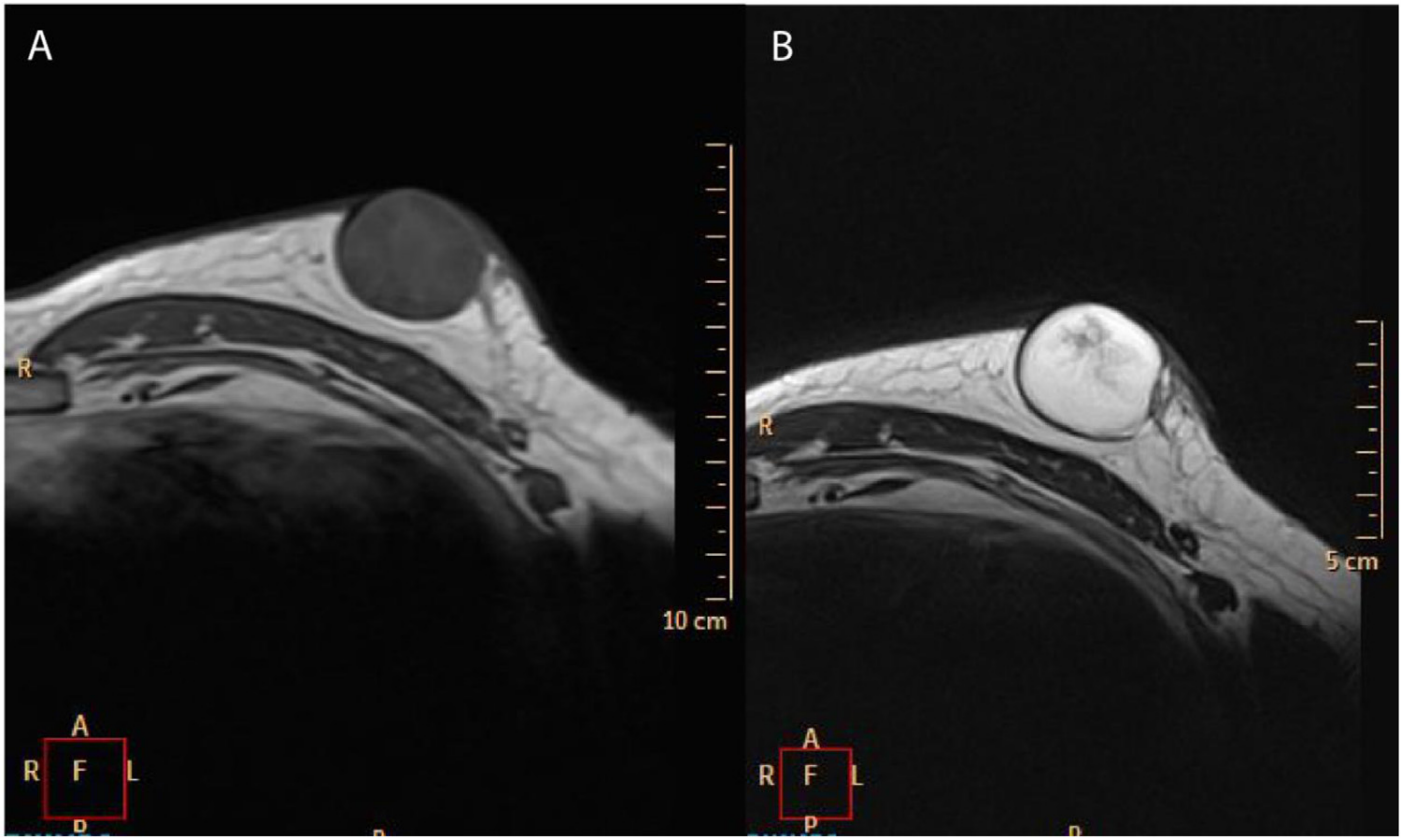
On magnetic resonance imaging (MRI); (a) lesion appeared mild hypointense on T1-weighted imaging, (b) hyperintense on T2-weighted imaging.
